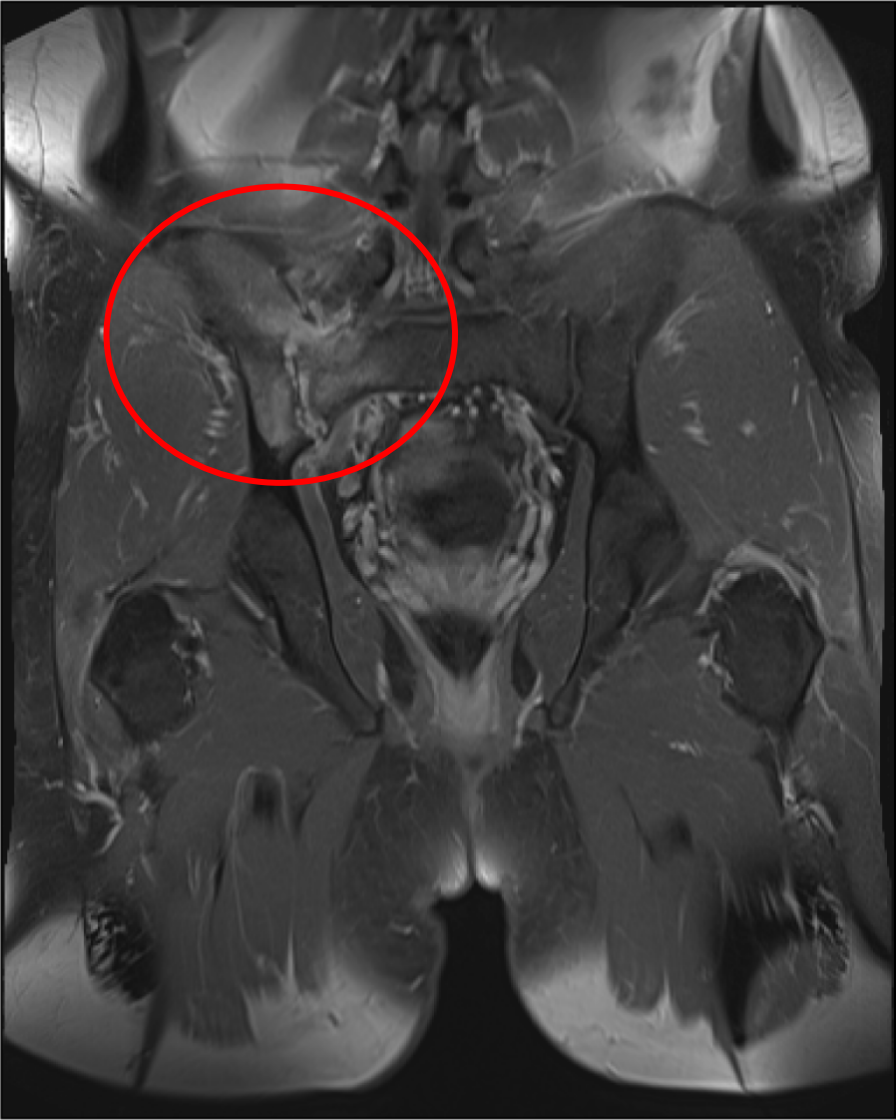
Sagittal T1, sagittal T2, axial T2, with addition of sagittal STIR and resolve sequences. MRI demonstrated small amount of fluid in the right SI joint with associated bone marrow edema suggesting sacroiliitis and osteomyelitis (circled)
